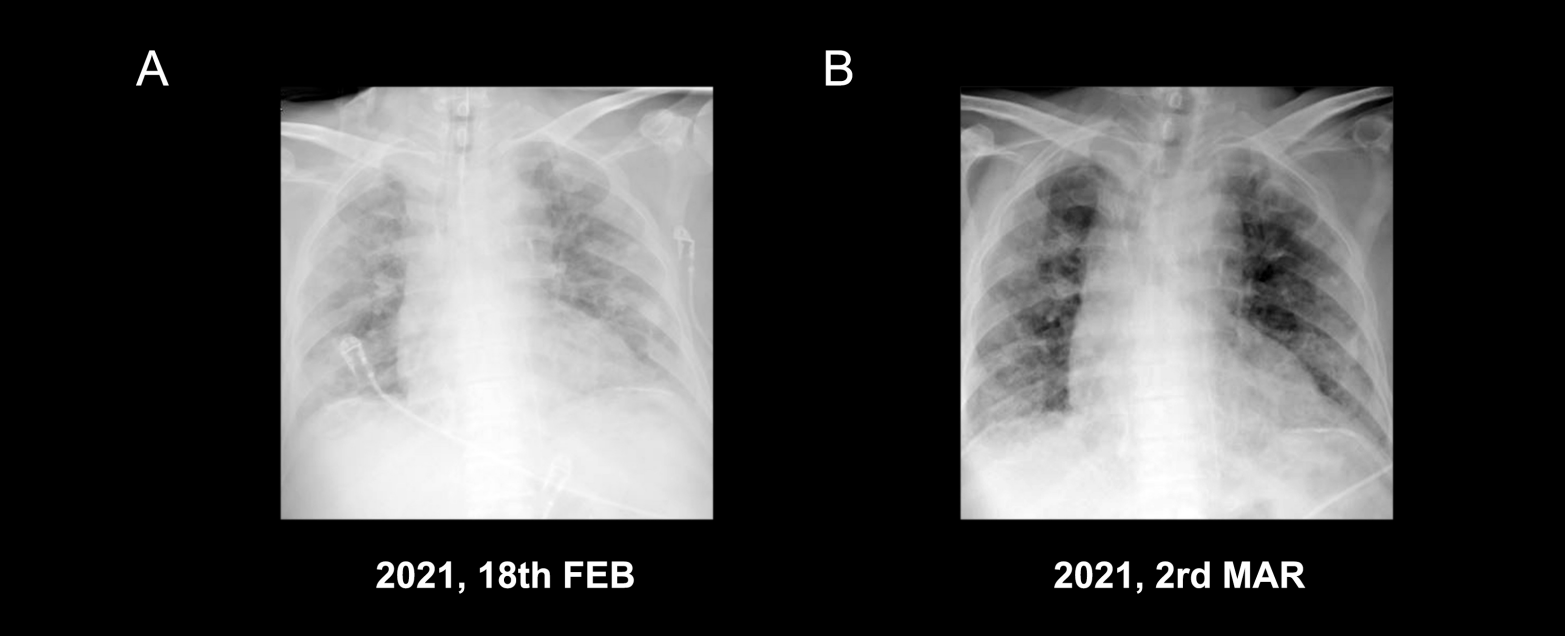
Bedside chest radiograph: both lung translucency and consolidation shadow decreased after treatment (A, B).
